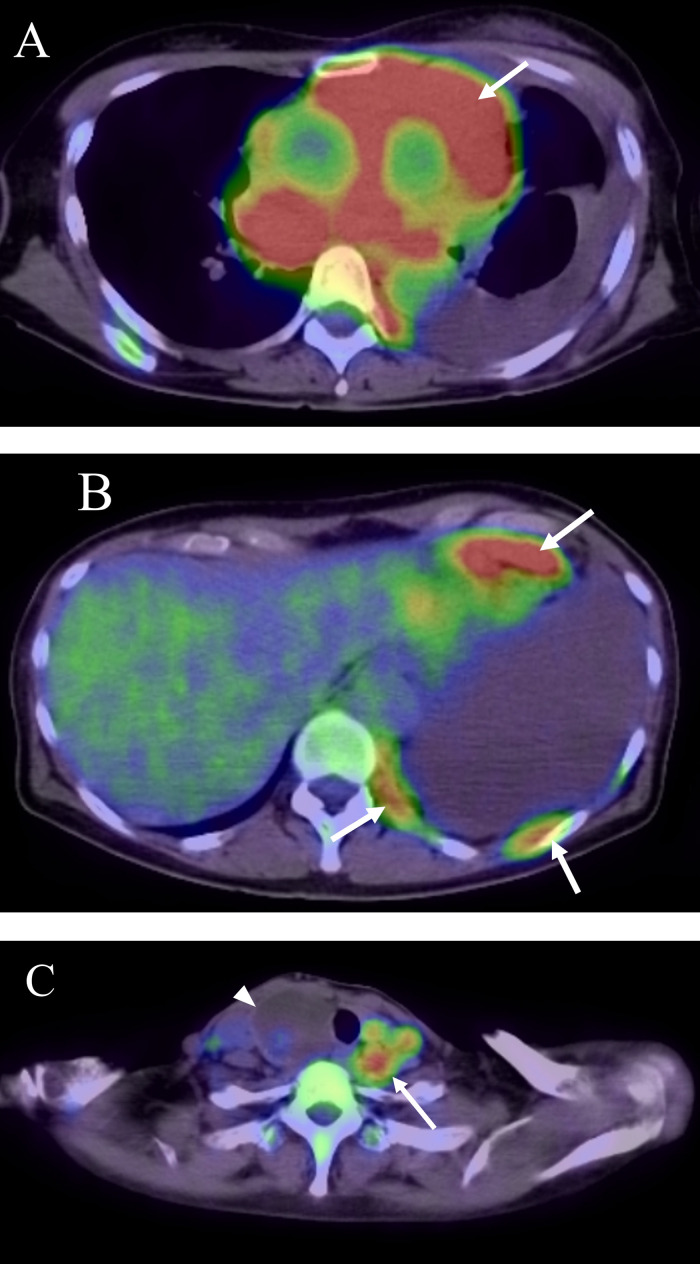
FDG PET-CT scan in the first case. FDG PET-CT scan showing strong uptake (SUV max = 9-12) in the mediastinal mass (arrow in A) along with nodular and linear uptake along the left chest wall (arrow in B). Multiple nodular uptake above the left clavicle (SUV max = 4-6) (arrow in C). Arrow head in C shows a benign thyroid tumor.
